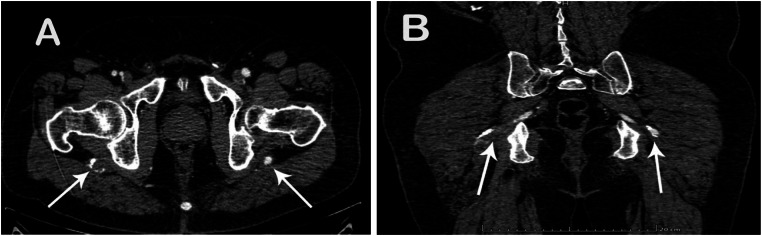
The Pelvic CTA of axial (A) and coronal (B) planes shows bilateral persistent sciatic artery (arrows) with calcified plaque and significant intraluminal thrombosis in right side.
